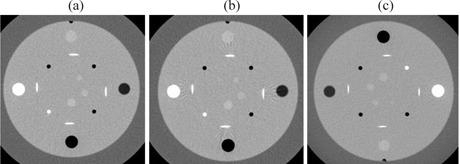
GE 16‐channel CT comparison, 2.5 mm thick images, for detector configuration: 16×1.25 mm. GE LS RT16, pitch 0.562 (a), pitch 1.375 (b), and GE LS16, pitch 1.375 (c).
